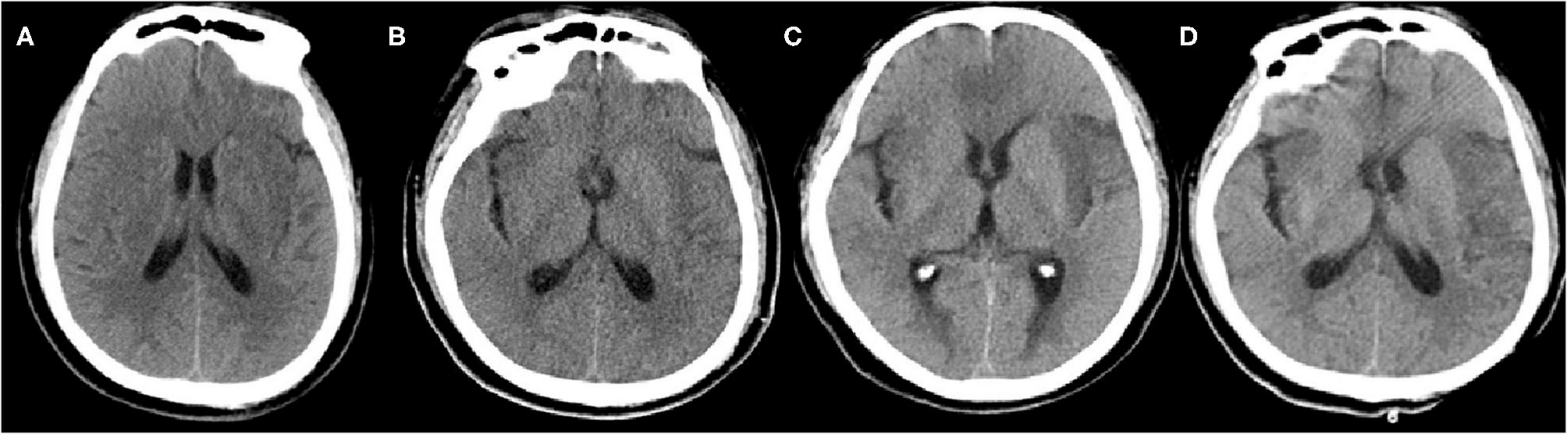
The plain CT brain imaging on the 8th day (A), 15th day (B), 19th day (C), and 24th day (D) after initial symptoms.
